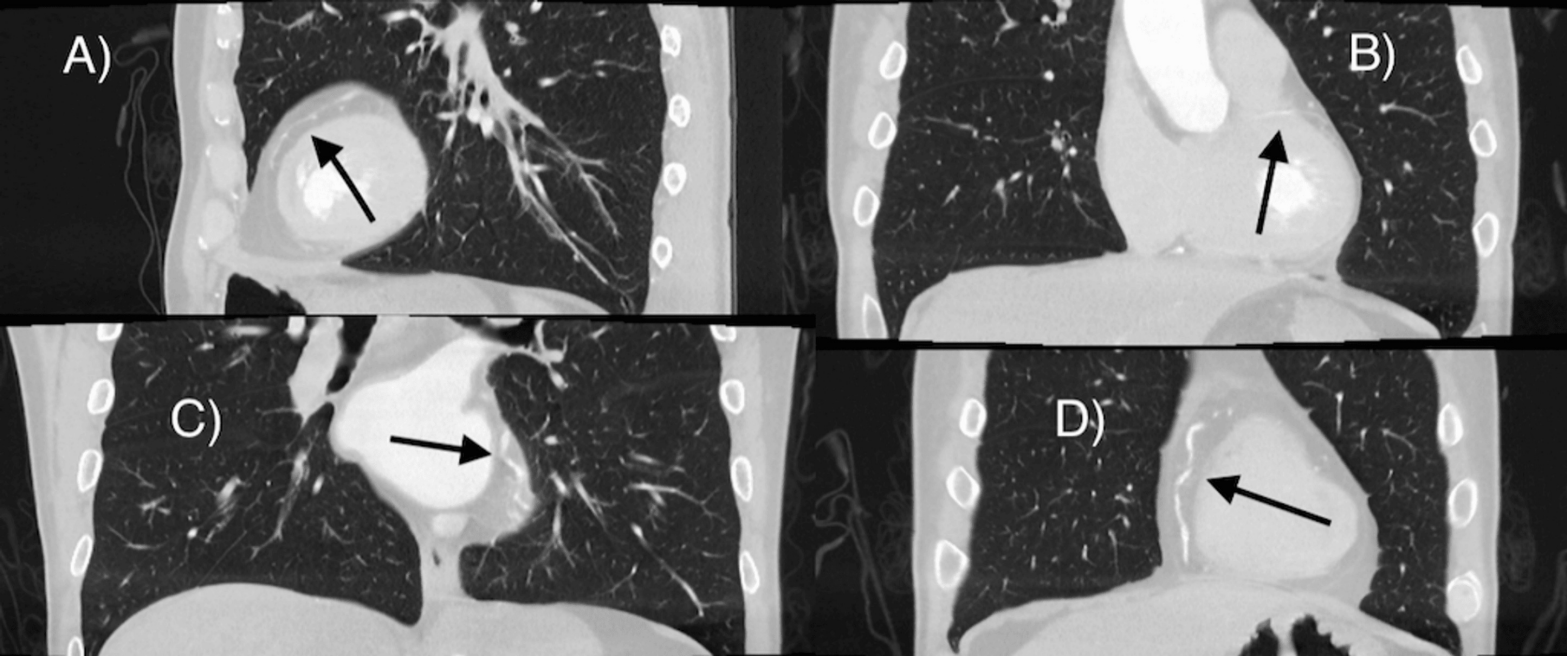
Computed tomography angiography of the heart and arteries. A) Anterior pathway of the left anterior descending artery (LAD) with septal branches running inferiorly. B) Marginal branch with mild proximal stenosis running laterally and then inferiorly. C) Left circumfle (LCX) is running inferolateral and originates from the left coronary cusp. D) Right coronary artery (RCA) running separately along the medial aspect of the heart with minimal stenosis.
